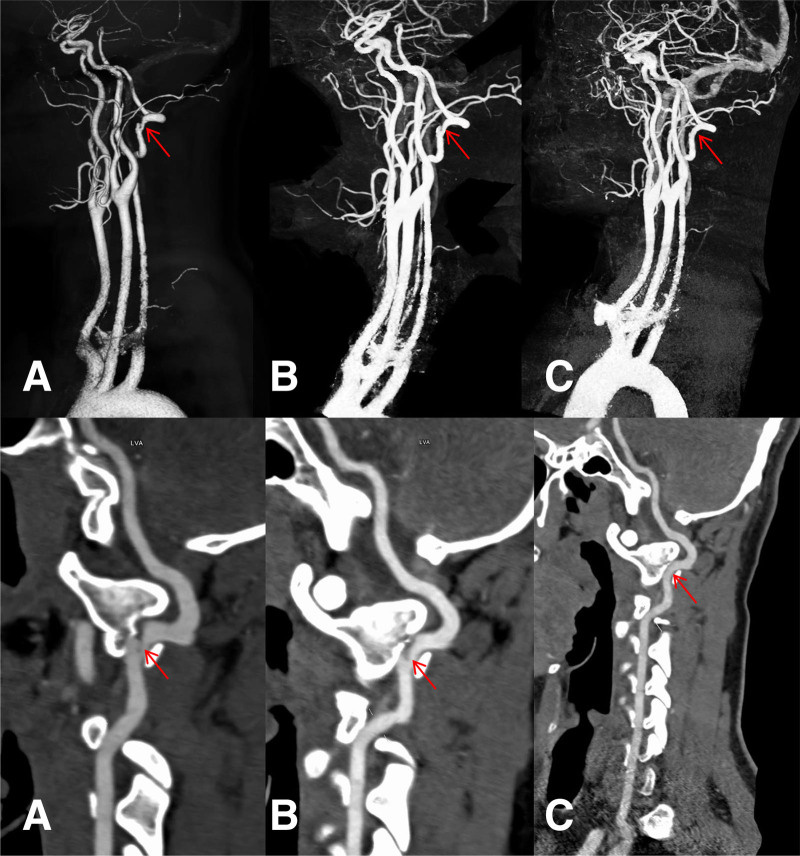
Images of cranial CTA at different times (arrows indicate anatomical location). (A) On January 24, 2019, CTA of the head and neck revealed moderate to severe stenosis and dissecting aneurysm formation in the lumen of the V3 segment of the left vertebral artery. (B) On October 1, 2019, CTA of the head and neck showed moderate to severe stenosis and dissecting aneurysm formation in the lumen of the V3 segment of the left vertebral artery. (C) On January 24, 2019, CTA of the head and neck showed complete repair of the left vertebral artery, with near-complete regression of the dissecting aneurysm. CTA = computed tomography angiography.
